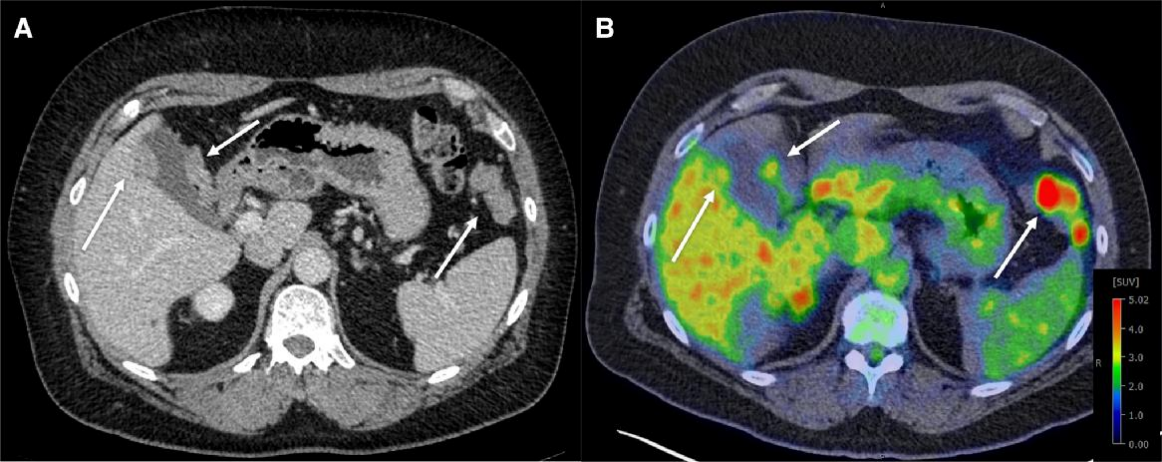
Contrast-enhanced axial CT from 2022 demonstrates enhancing soft-tissue nodules in the left upper quadrant measuring up to 6.7 cm and associated with the gallbladder wall (arrows) and right subdiaphragmatic and periportal nodules A). Axial fused ^18^fluorine fluorodeoxyglucose positron emission tomography-computed tomography demonstrates mild to moderate avidity in the left upper quadrant mass (SUVmax, 9.8) and in the gallbladder wall lesions (SUVmax, 4.2).
